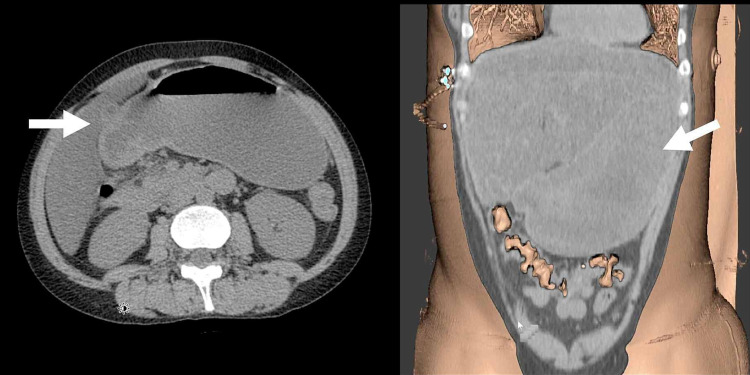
Sagittal and coronal CT imaging demonstrates gastric outlet obstruction with dilatation of the stomach and distal esophagus (see arrows).
